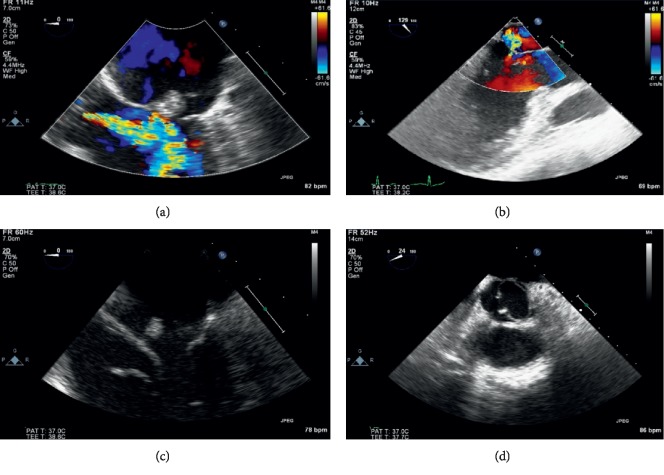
This demonstrates a transesophageal echocardiogram for evaluation of endocarditis. (a): Mid esophageal view showing a large vegetation on the anterior leaflet of the mitral valve. The color doppler shows severe aortic regurgitation jet towards the anterior mitral leaftet. (b): Mid esophageal long axis view demonstrating severe mitral regurgitation. (c): Mobile echo density on the atria aspect of the mitral valve. (d): Aortic valve is found to be a bicuspid valve associated with severe aortic regurgitation.
